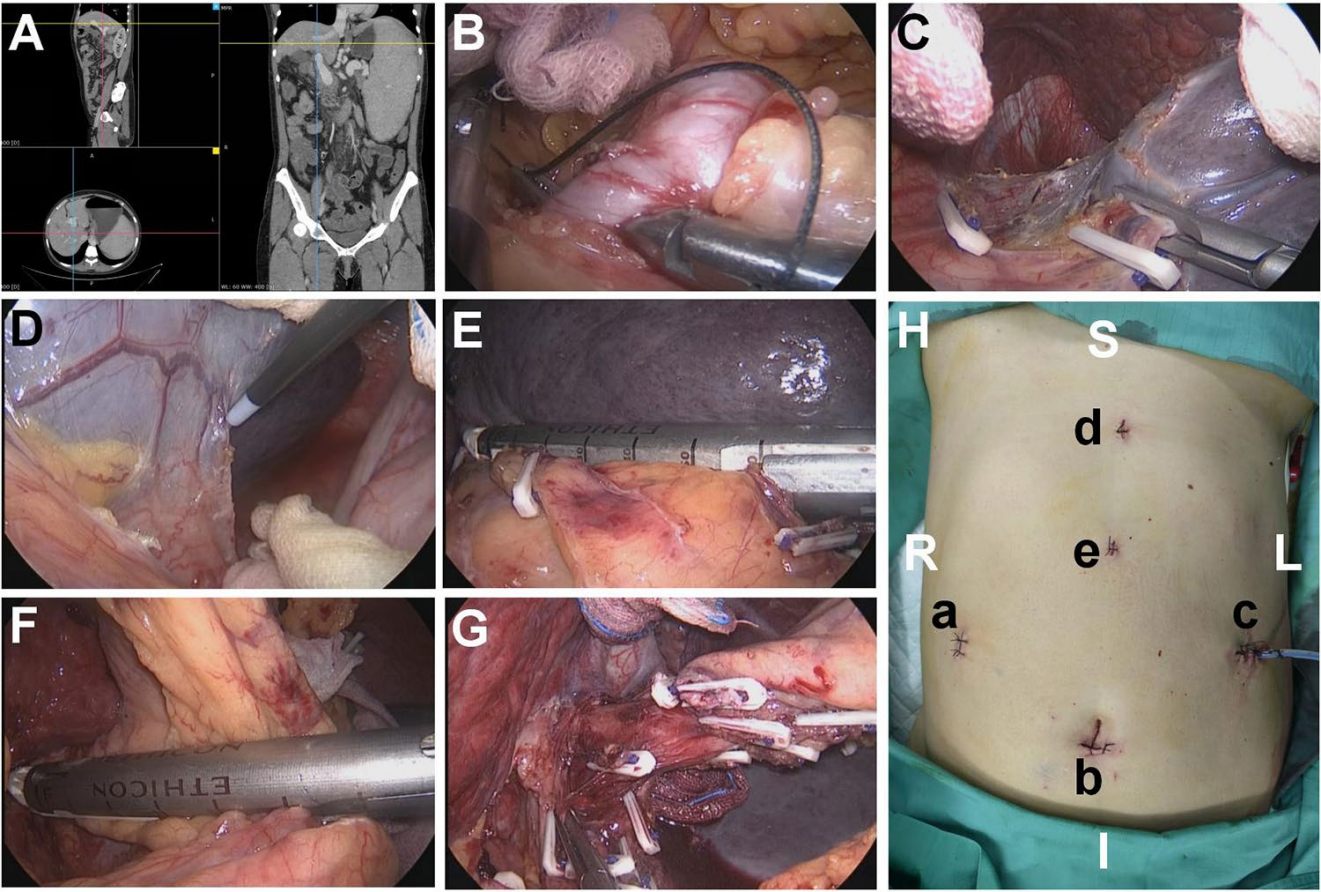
Evaluation and main steps in laparoscopic splenectomy and esophagogastric devascularization procedure (showed with the same patient). A Preoperative abdominal enhanced CT evaluation; B the splenic artery separation and ligation; C short gastric vessels dissection by absorbable hemostatic clamps; D the dorsal splenic ligament separation; E splenic hilar pedicles transection with the Endo GIA™ stapler; F the left gastric vein transection with the Endo GIA™ stapler; G esophagogastric devascularization by absorbable hemostatic clamps; H laparoscopic surgery completion with an abdominal drain. Trocar locations: laparoscopic observation hole (a); Main operative holes (b, c); Assistant operative holes (d, e). *S* superior, *I* inferior, *R* right, *L* left
